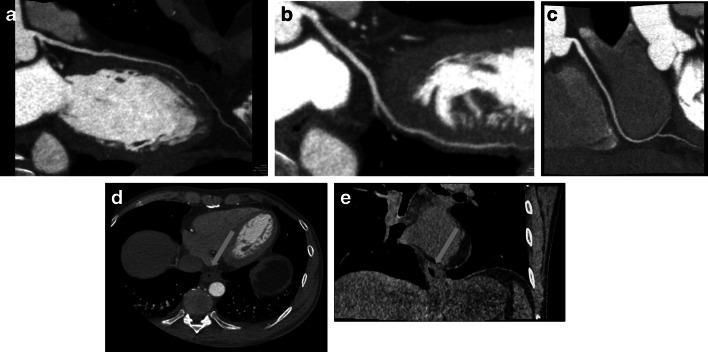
Forty-eight-year-old man with a 3.7 × 4.0 cm esophageal hiatal hernia (*arrow*), which after gastroenterological consultation and initiation of acid blocker treatment turned out to be a potential cause of chest pain in this patient. CTA detected no significant coronary artery stenoses in this patient. a LMA and LAD, curved reconstruction. b LCX, curved reconstruction. c RCA curved reconstruction. d Soft tissue reconstruction, axial plane. e Soft tissue reconstruction, coronal plane
